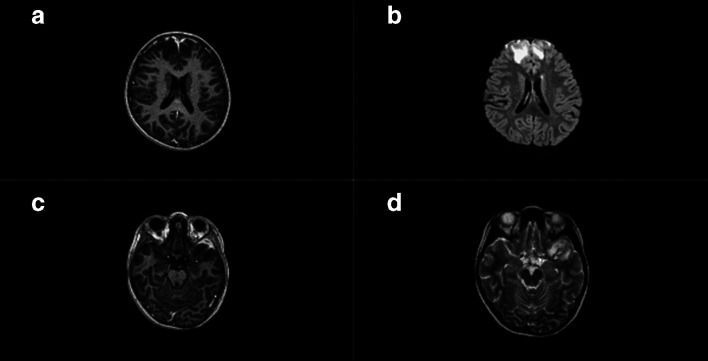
MRI imaging. (a) T1 gado: hypo intensity bilateral frontal with leptomeningeal enhancement. (b) FLAIR bilateral hyperintensity (vasogenic edema) in white matter and in subarachnoid space. (c) T1 gadolinium: enhancement of leptomeningeal with small empyema left middle cranial fossa. (d) T2 C involvement, left temporal lobe.
